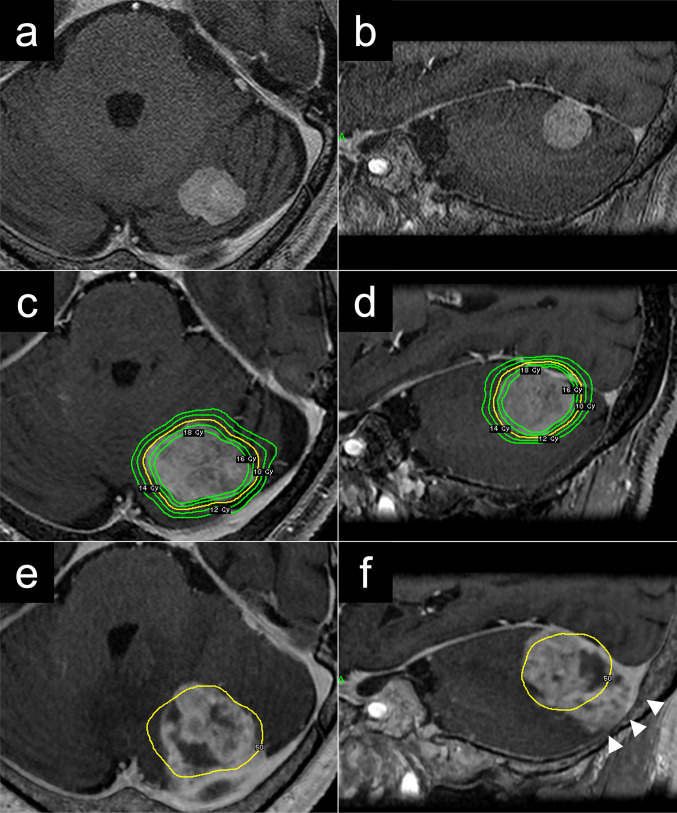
Gadolinium-enhanced T1-weighted magnetic resonance imaging at initial presentation, before, and after stereotactic radiosurgery (SRS). Axial and sagittal images at initial presentation show a 17-mm enhancing tumor at the cerebellar tentorium (a, b). Over 16 years, the tumor gradually grew to 33 mm by age 70. SRS was performed with a prescription dose of 14 Gy prescribed to the 50% isodose line (c, d). Follow-up images obtained 37 months after SRS show the controlled anterior irradiated tumor (yellow circle, 14 Gy to the 50% isodose line) and a newly developed posterior lesion (white arrowhead) (e, f)
